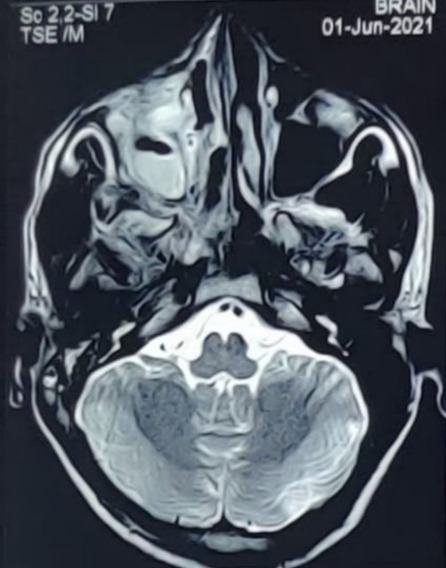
MRI axial section showing marked mucosal thickening of the right maxillary sinus
